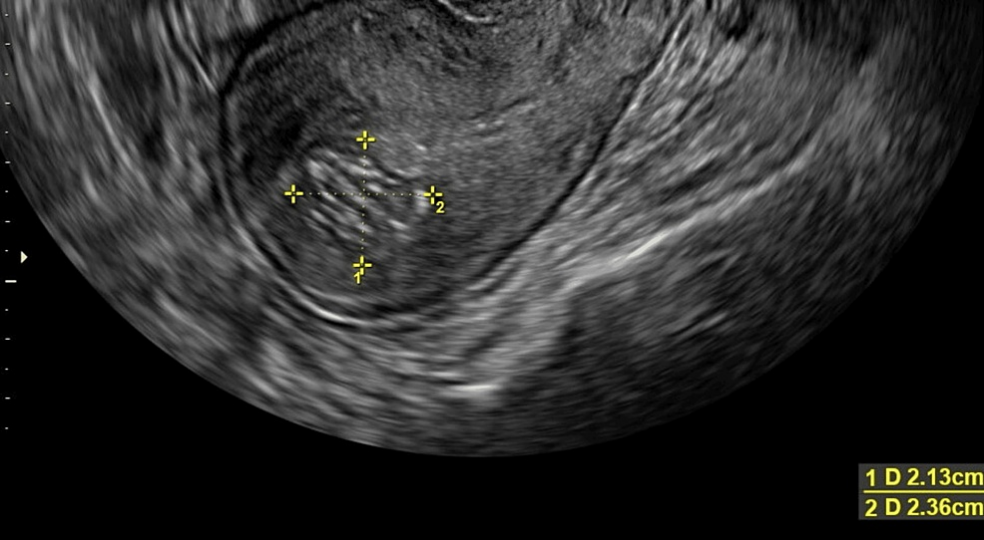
Ultrasound image showing a posterior intramural fibroid measuring 2.1 x 2.4 x 2.3 cm
